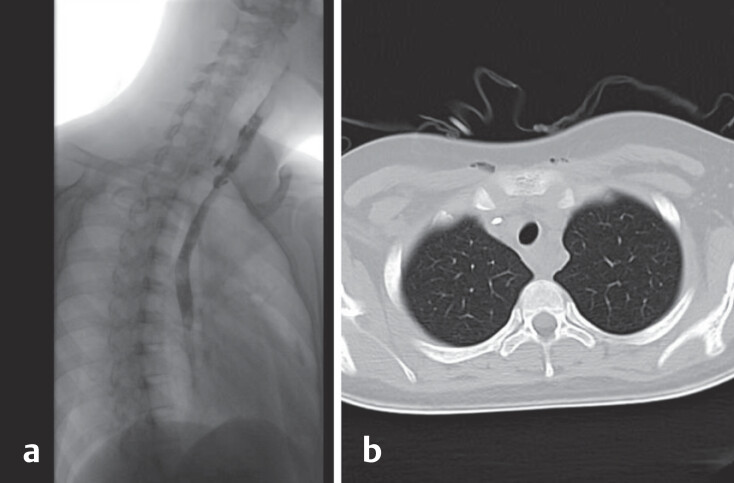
Repeat upper gastrointestinal radiography and computed
